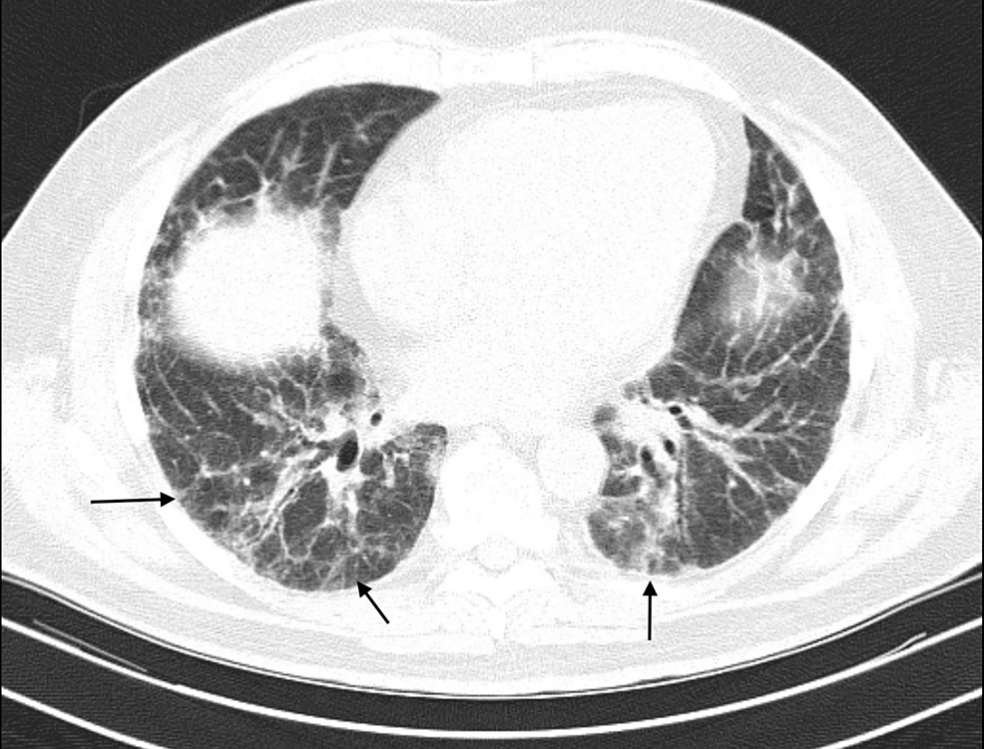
High-resolution computed tomography of lung bases showing subpleural reticulation more prominent posteriorly. Long arrow: reticulation
